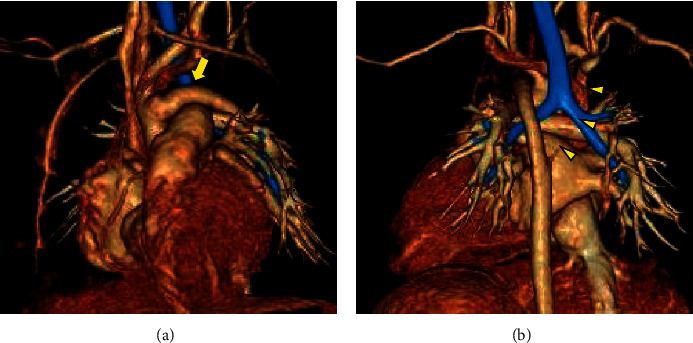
(a, b) Contrast-enhanced three-dimensional CT images obtained 21 days after surgery. (a) The front view and (b) the rear view. There is no stenosis in the reconstructed left aortic arch (yellow arrow) and the right distal arch is regressed (small yellow arrowheads).
